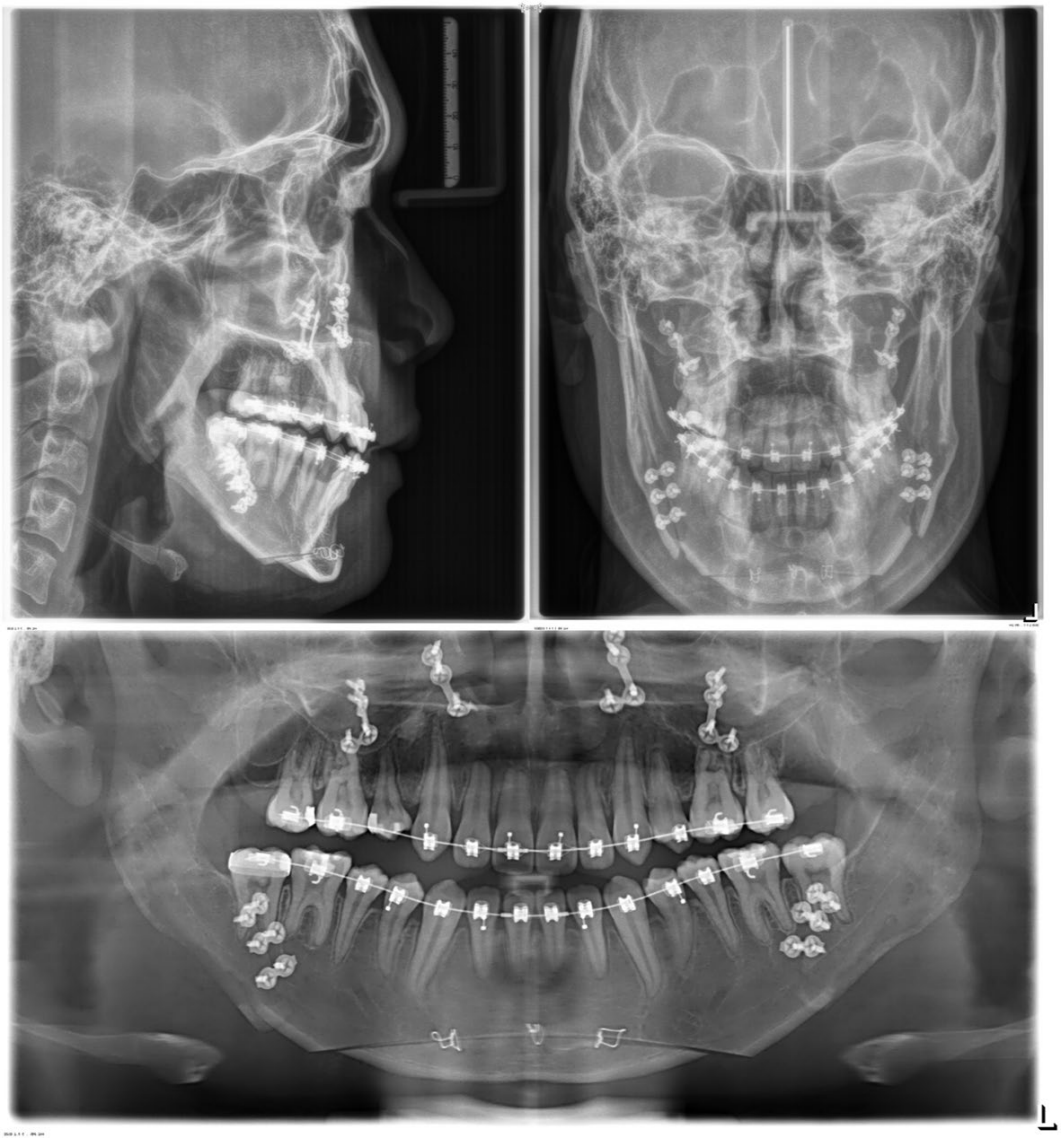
Post-surgical radiographs after two-jaw orthognathic surgery
